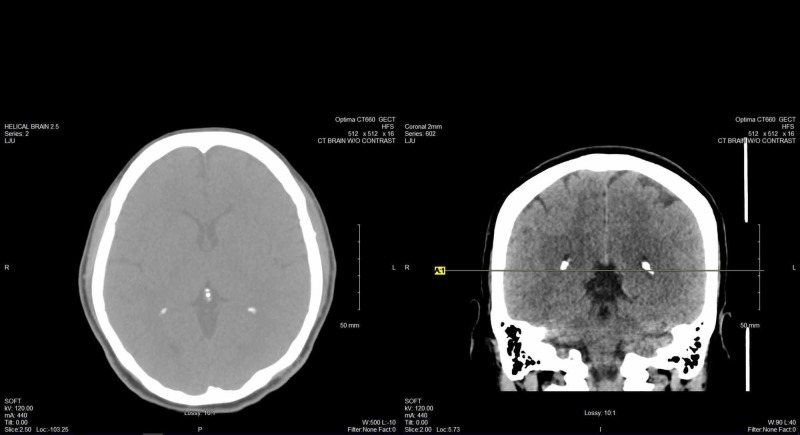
Axial and coronal views of CT brain without contrast, highlighting the periventricular white matter hypodensities CT: computed tomography
